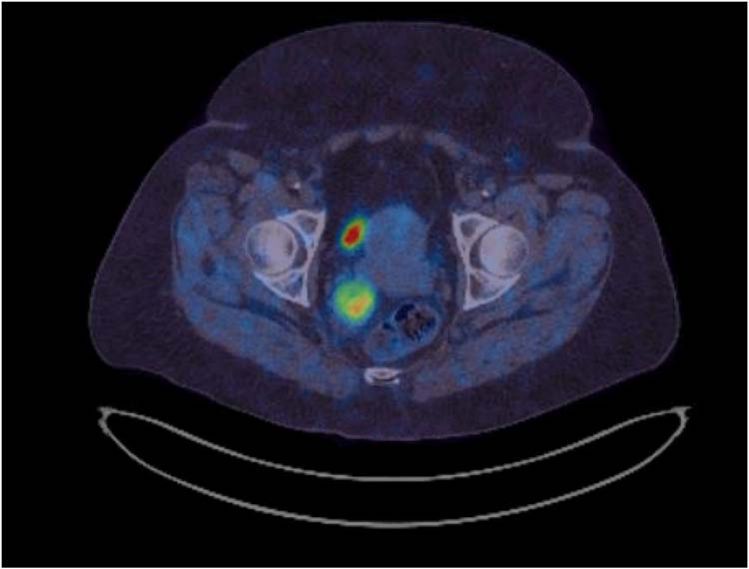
Hypersignal on Gallium-68 Dotatate PET scan (SUVmax 9.4).
